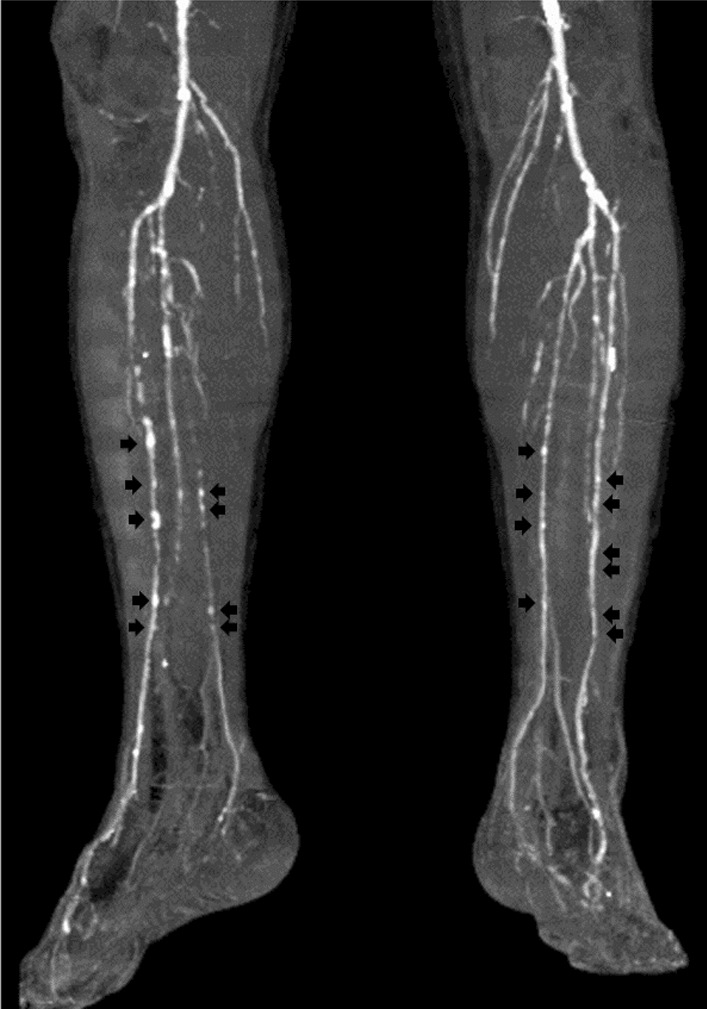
Computed tomography of the lower legs showing spotty calcification in the bilateral infrapopliteal arteries (black arrows)
